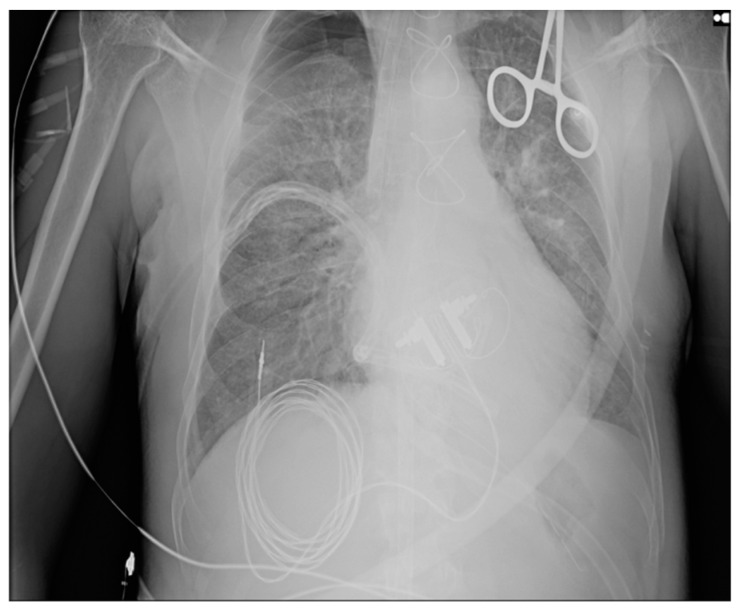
Chest X-ray suggestive of ARDS.
